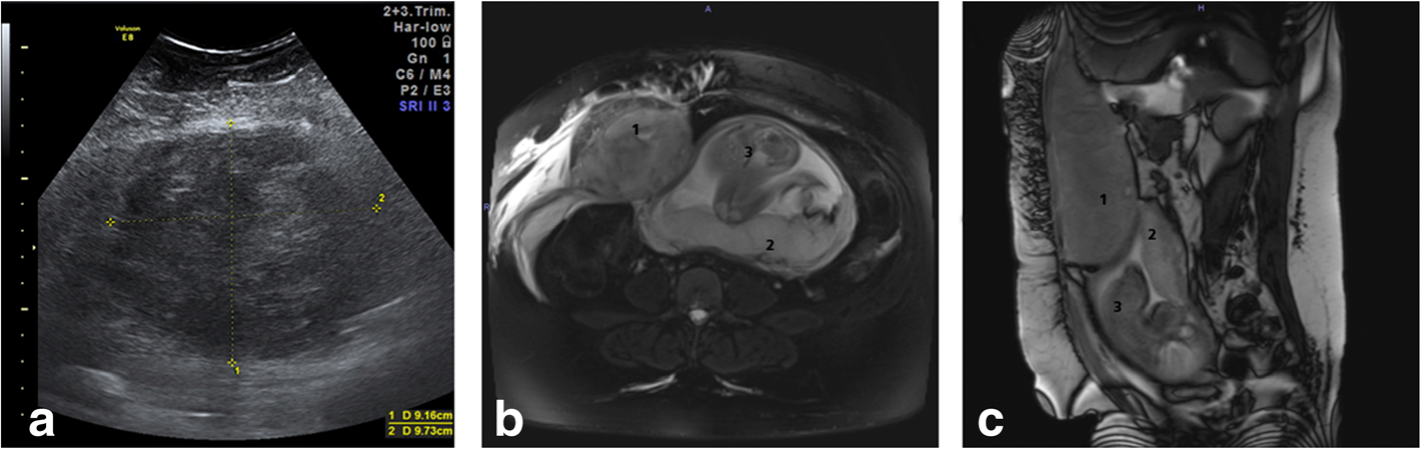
a A 26-year-old pregnant woman at 27 weeks’ gestation presented with right-sided abdominal pain. Transversal transabdominal ultrasound of her right lumbar region showed a 9.16 × 9.73 cm, round, well-demarcated and partly hypoechoic/isoechoic structure, appearing to be in contact with her uterine wall. b Magnetic resonance imaging of her abdomen, coronal view: Type III rectus sheath hematoma (*1*) measuring 11 × 12 × 20 cm, mainly located at the right side and starting to displace her uterine wall. The placenta (*2*) is located at the rear aspect of her uterus and appears to be normal, without sign of acute abruptio placentae. c Magnetic resonance imaging of her abdomen, axial view: The fetus (*3*) is lying in cephalic presentation. Note the associated edema of the subcutaneous tissue
